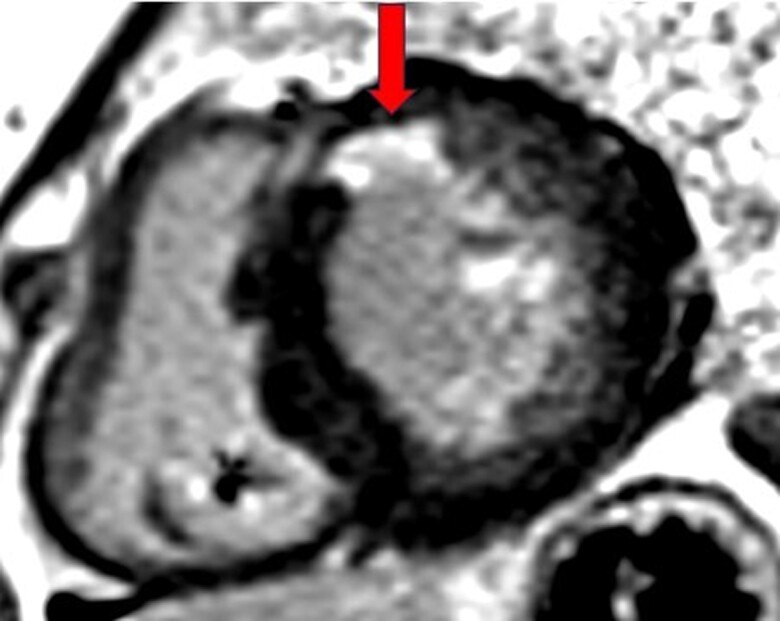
Post-contrast cardiac magnetic resonance imaging revealing subendocardial late gadolinium enhancement of the anterior basal myocardial segments in basal short-axis view (red arrow).
